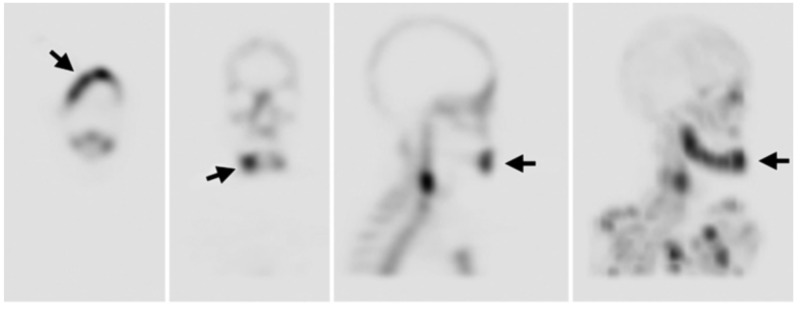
SPECT bone scintigraphy SPECT bone scintigraphy shows increased uptake in the right mandible (black arrows) SPECT: single-photon emission tomography
